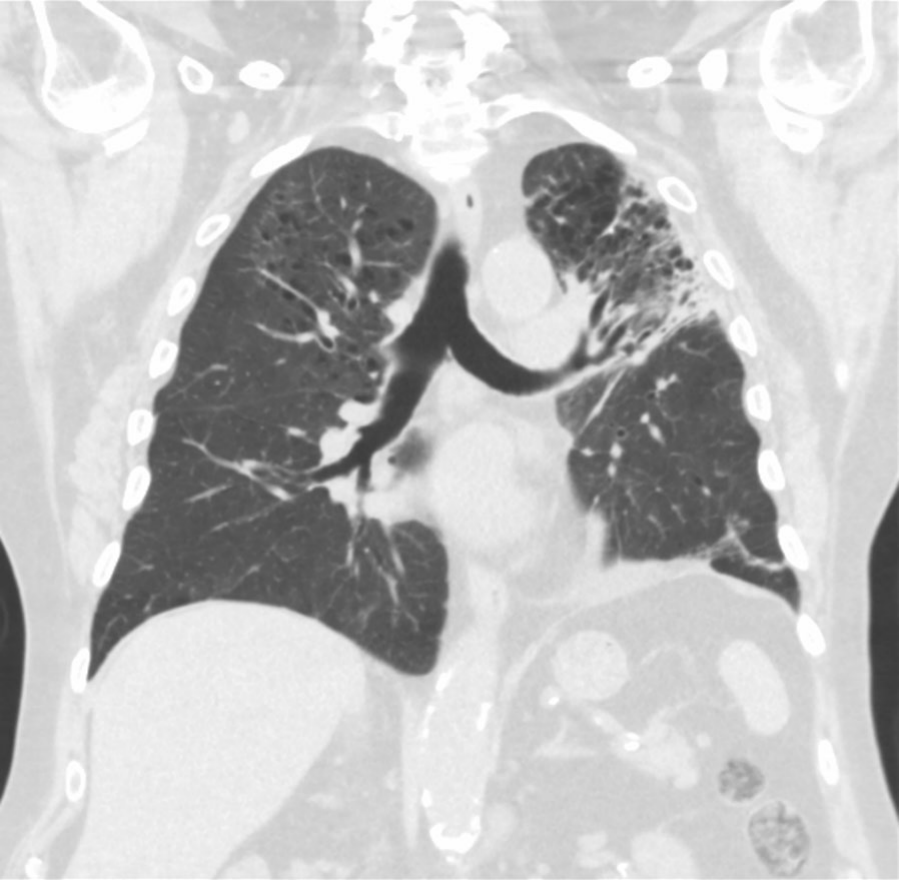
Case 3—CT chest after treatment of pneumonitis with initial recovery
